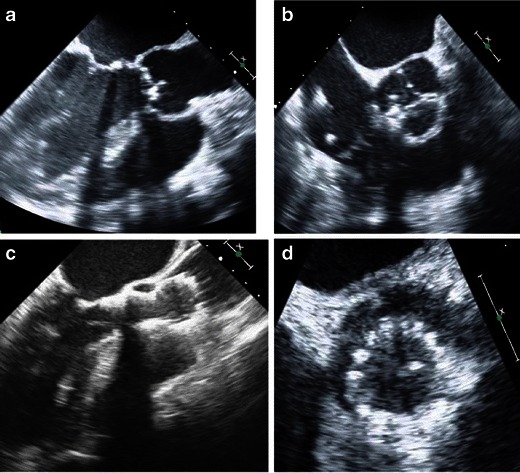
Intraprocedural baseline transesophageal echocardiography and echocardiographic imaging with the CoreValve THV in situ. a Long-axis view showing heavily stenosed aortic valve. b Short-axis imaging showing a calcified and stenosed aortic valve in systole with poor leaflet opening and small aortic valve opening area. c Transesophageal long-axis view showing the CoreValve THV frame with the inflow portion positioned in the left ventricular outflow tract/aortic annulus and the outflow (wider frame) within the aortic root/proximal ascending aorta. d Transesophageal short-axis view showing inflow of the device frame in position within the left ventricular outflow tract
